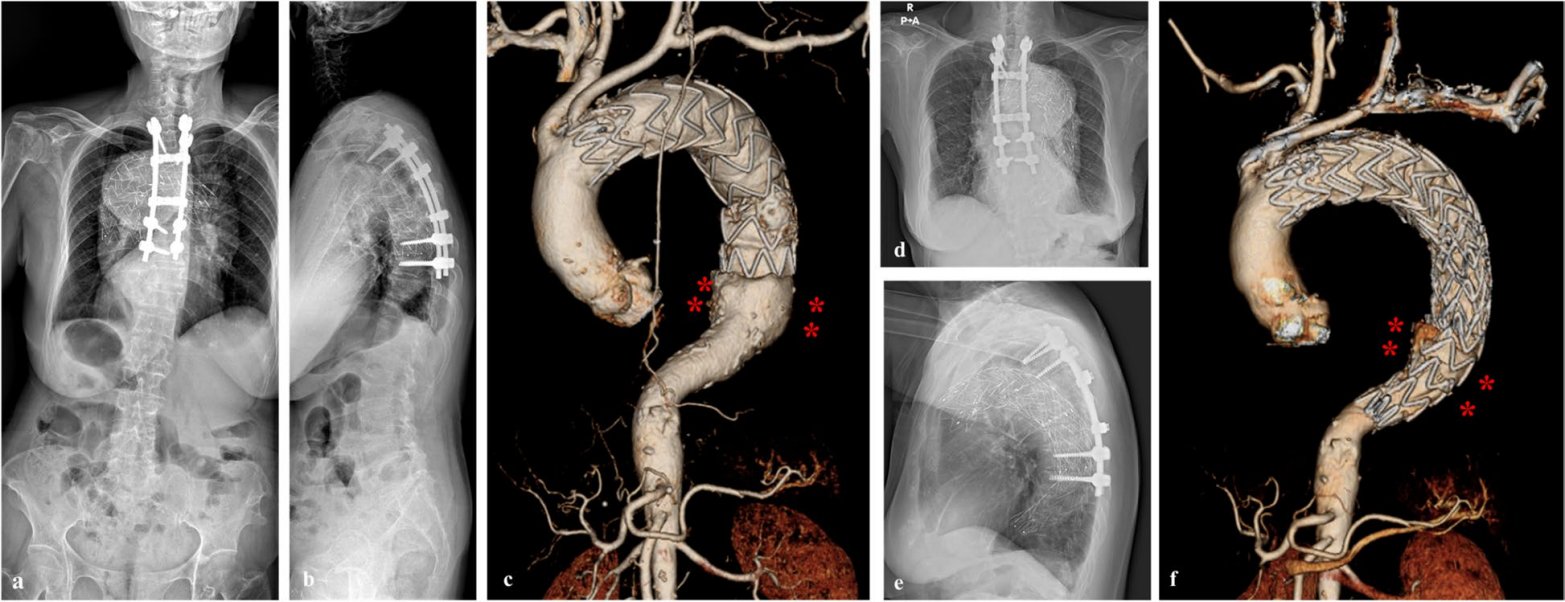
Surgical treatment in this case. a and b, Considering the rapid progression of this disease, posterior instrumentation and fusion from T3–T9 were performed to support the stability of the thoracic spine. c, Follow-up computed tomography of the aorta after posterior fusion showed remnants of the perianeurysmal outpouching lesion from endo-leakage after thoracic endovascular aortic repair (TEVAR). d–f, TEVAR was performed and significantly decreased the lesion remnants
